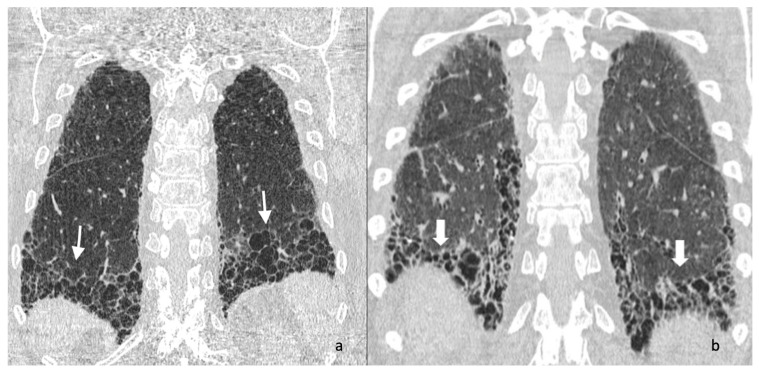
Coronal HRCT images of the chest showing the “straight edge sign” (white arrows in (a,b), characterized by sharp demarcation of fibrosis with a straight, horizontal lower boundary, limited to the lung bases without significant extension along the lateral margins. This imaging feature is often associated with connective tissue disease-related interstitial lung disease (CTD-ILD) and helps differentiate it from idiopathic pulmonary fibrosis (IPF).
